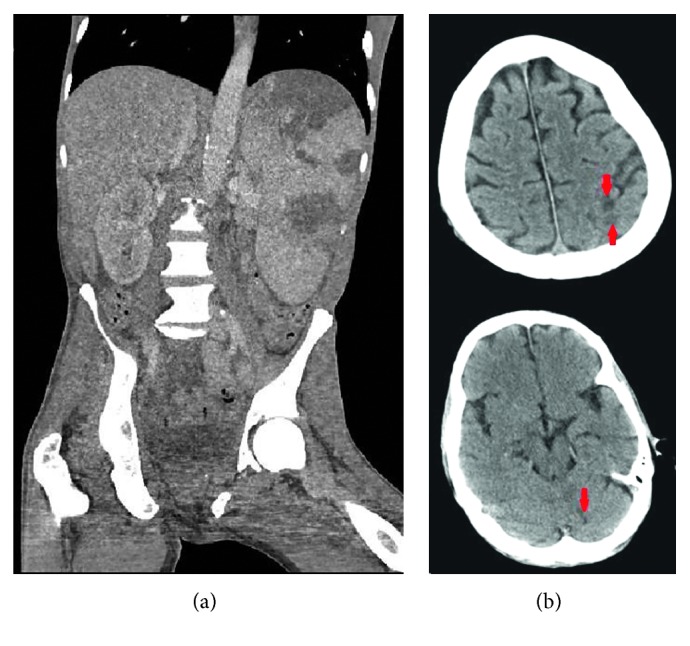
(a) Computed Tomography (CT) of the abdomen and the pelvis without contrast showing large wedge-shaped splenic infarcts. (b) CT of head without contrast displaying hypodensities in the left parietal lobe and left cerebellum due to septic emboli, shown in red arrows.
